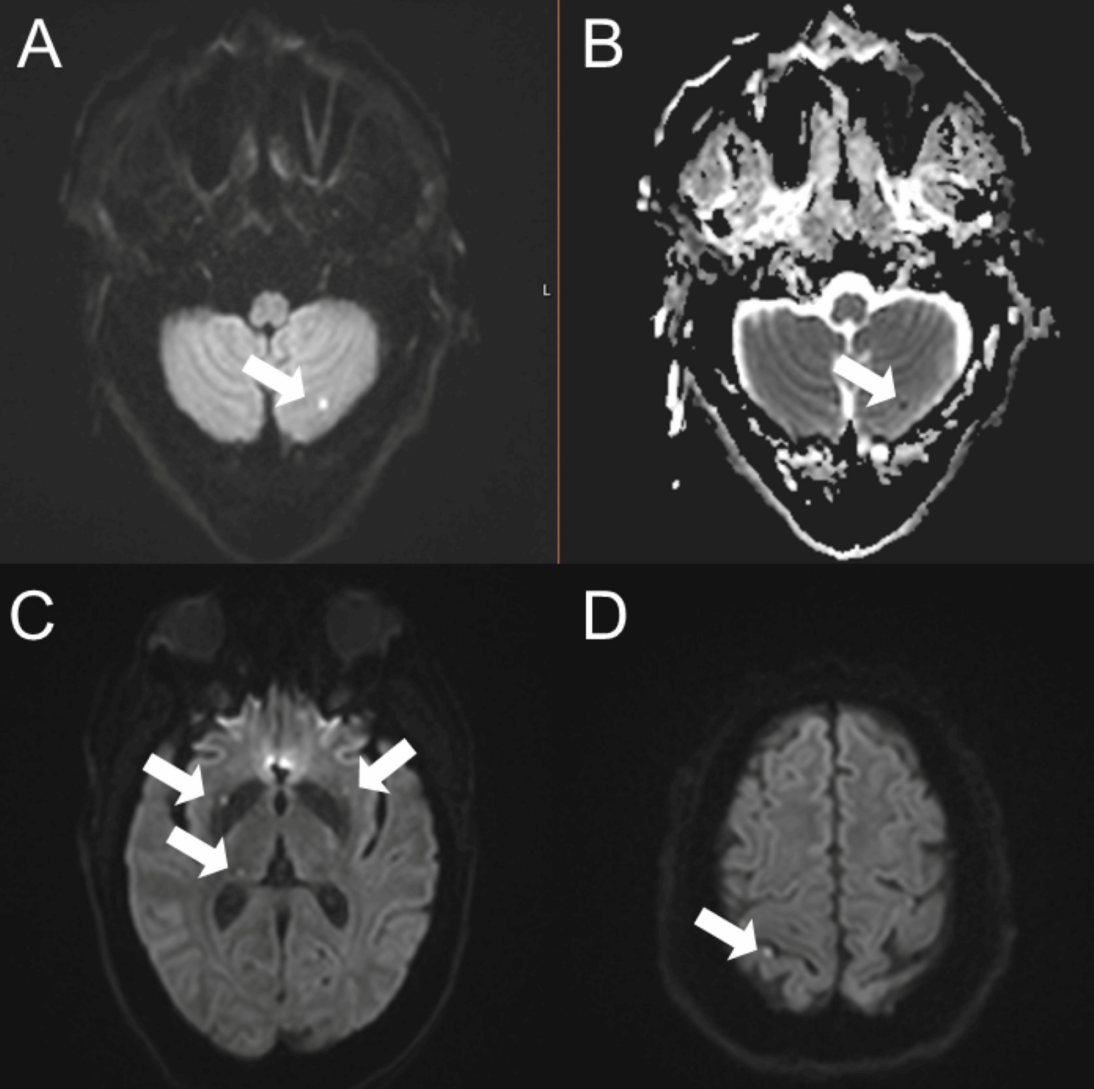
Brain MRI showed numerous acute punctate infarcts consistent with a multiterritorial ischemic stroke. Axial diffusion-weighted imaging (A) reveals focal diffusion restriction in the left cerebellum (arrows), confirmed by true restricted diffusion on the corresponding ADC map (B). Additional DWI images demonstrate infarcts in the basal ganglia and thalami (C), as well as the frontal lobes (D). ADC: Apparent diffusion coefficient, DWI: Diffusion-weighted imaging
